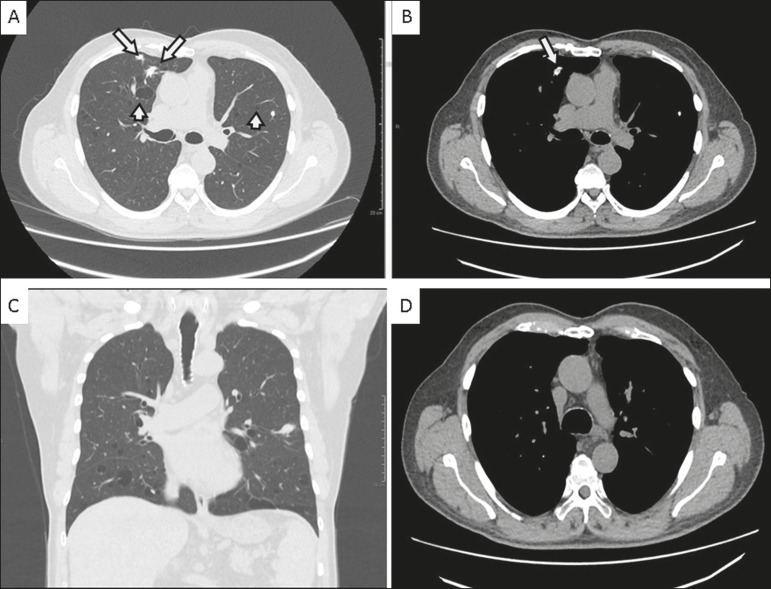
A,B: Axial CT images of the chest at the level of the main bronchi, with lung and mediastinal window settings (A and B, respectively), showing thin-walled cysts in the peribronchovascular and subpleural regions (short arrows), as well as irregular, calcified pulmonary nodules (long arrows). Note also the preservation of the thickness of the bronchial wall. C: Coronal CT scan of the chest, with lung window settings, showing sparse nodules and cystic predominance in the middle and lower lung fields. D: Axial CT scan of the chest, with mediastinal window settings, highlighting the absence of mediastinal lymphadenopathy and the preservation of the thickness of the tracheal wall.
